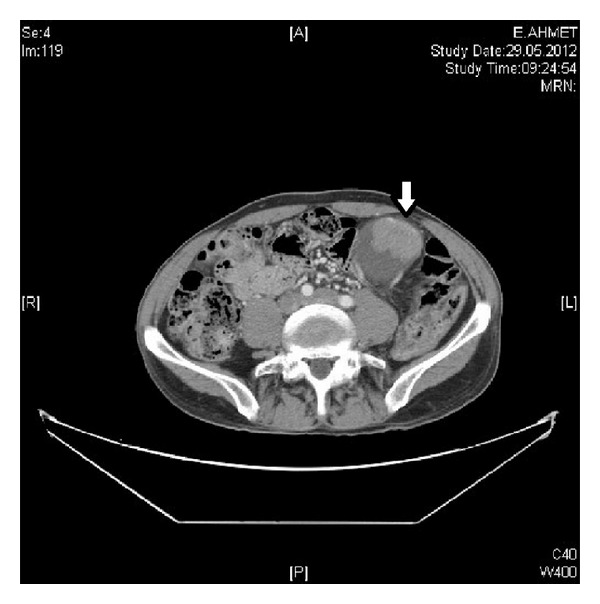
CT image of the solid mass in the left ureteropelvic junction with severe hydronephrosis.
